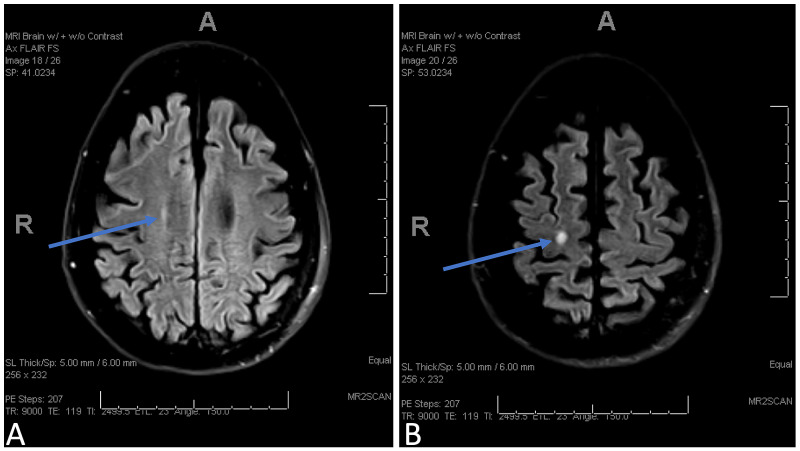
Axial FLAIR MRI. (A, B) Right centrum semi-ovale with hyperintense demyelinating lesion without enhancement (blue arrows). FLAIR, fluid-attenuated inversion recovery; MRI, magnetic resonance imaging
